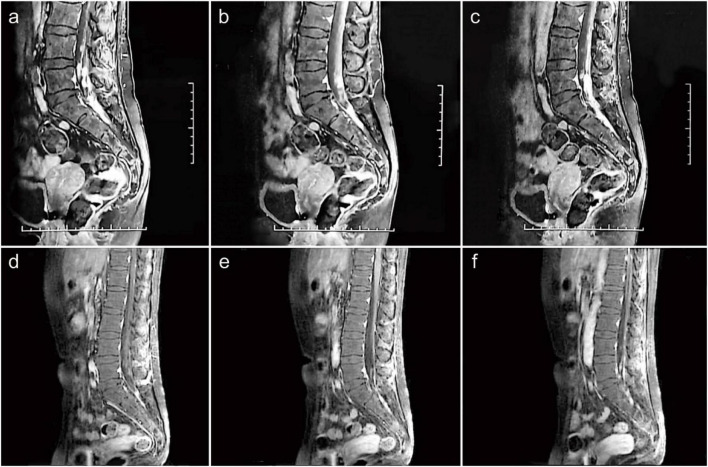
It shows that the lesions in the spinal cord of the lumbosacral region have significantly reduced and partially disappeared. (a–c) Shows MR images taken in an external hospital in July 2022, and (d–f) show those taken in our hospital in October 2022.
